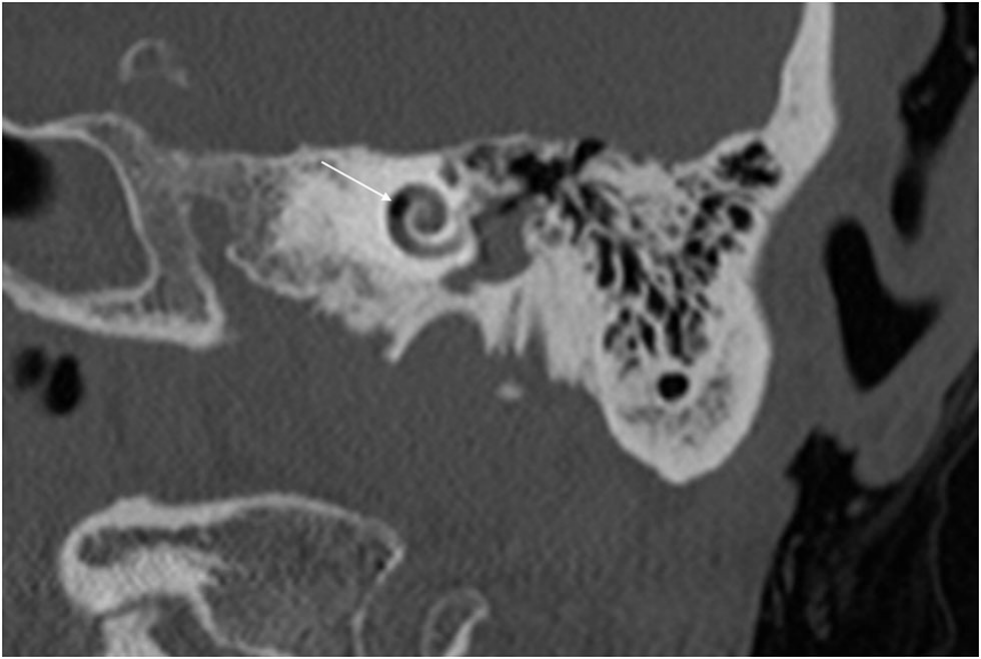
Coronal CT of temporal bone showing air in the second cochlear turn (arrow).
